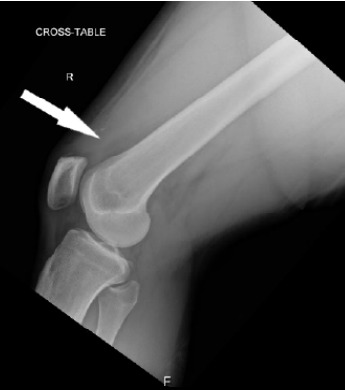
Plain radiograph of the right knee demonstrating trace suprapatellar joint fluid (arrow).
